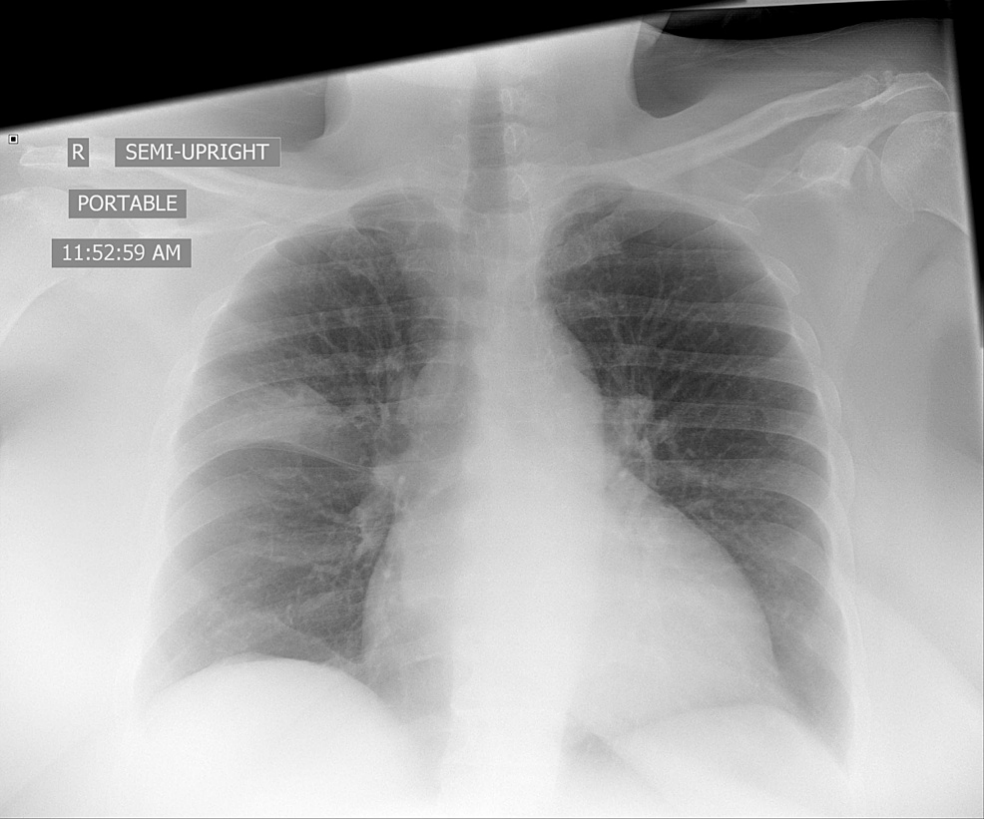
Focal right upper lobar opacity on chest X-ray
